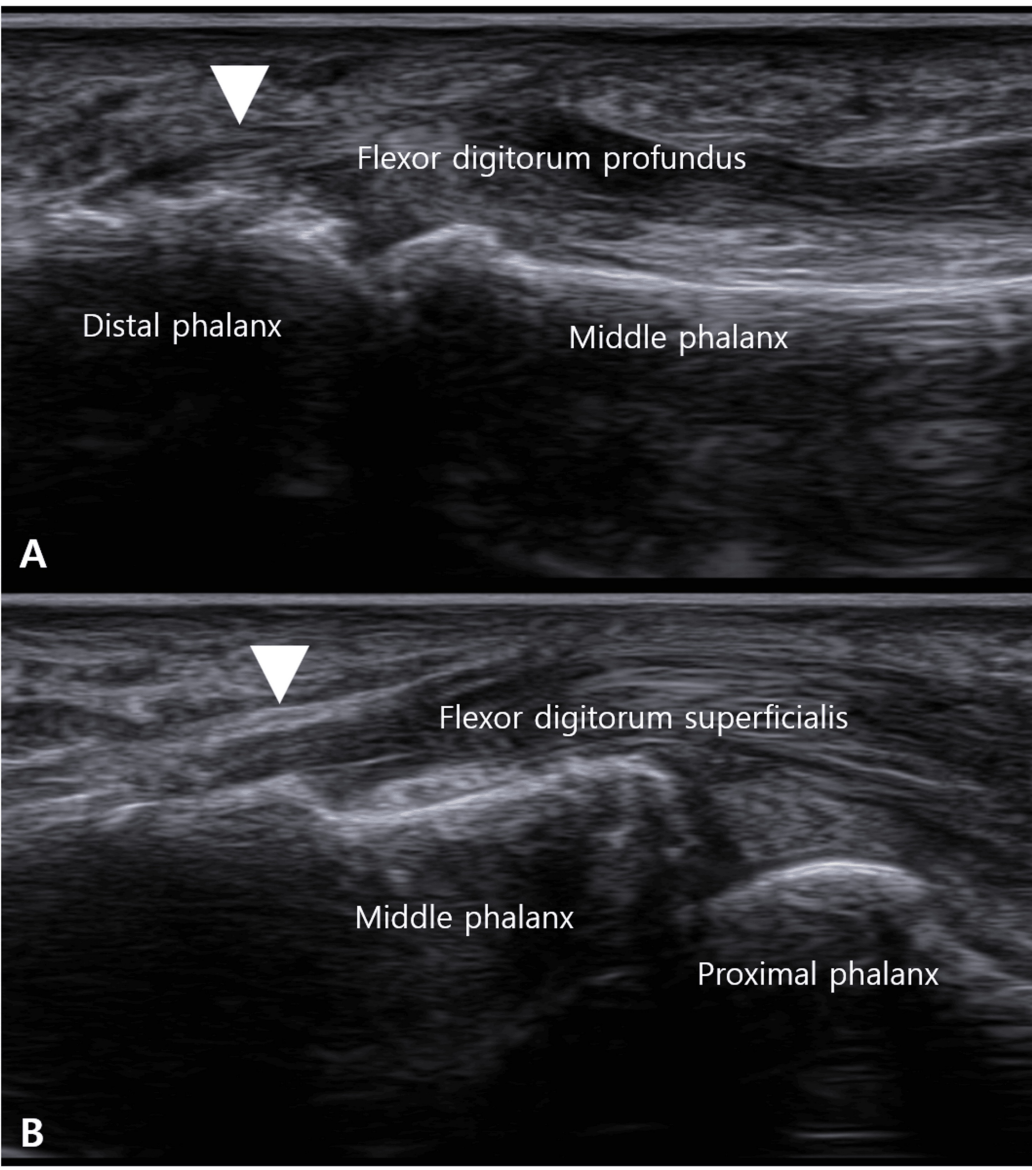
Ultrasound long-axis view showing palmar bony ridges (white arrowheads) at the attachment sites of the flexor digitorum profundus (A) and flexor digitorum superficialis (B) to the distal and middle phalanx.
